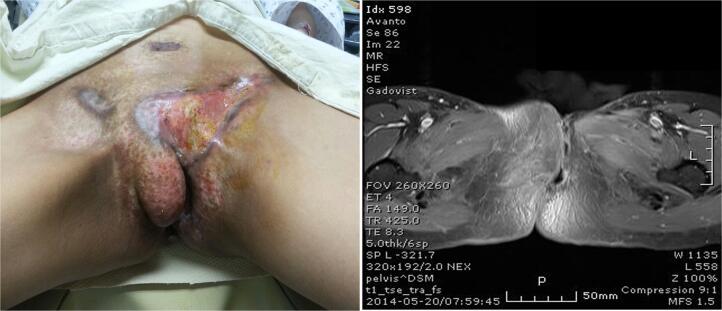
(a) Gross photos show significantly decreased vulvar lesion. (b) MRI shows partial resolution of vulvar lesion.
